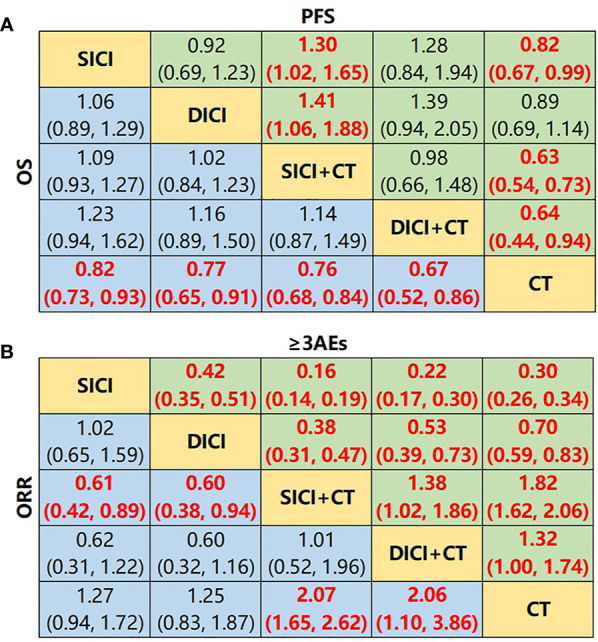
Network meta-analysis of SICI, DICI, SICI+CT, DICI+CT, and CT. (A) Pooled hazard ratio (HR) [95% CrIs (credible intervals)] for overall survival (OS) and progression-free survival (PFS) in the overall population. (B) Pooled odds ratio (OR) (95% CrIs) for objective response rate (ORR) and adverse events of grade 3 or higher (≥3AEs) in the overall population. Data in each cell are HR or OR (95% CrIs) for the comparison of upper row-defining treatment *vs*. lower row-defining treatment. HR less than 1 and OR more than 1 favor upper-row treatment. Significant results are highlighted in red and bold. SICI, single immune checkpoint inhibitor; DICI, double immune checkpoint inhibitors; SICI+CT, single immune checkpoint inhibitor combined with chemotherapy; DICI+CT, double immune checkpoint inhibitors combined with chemotherapy; CT, chemotherapy.
